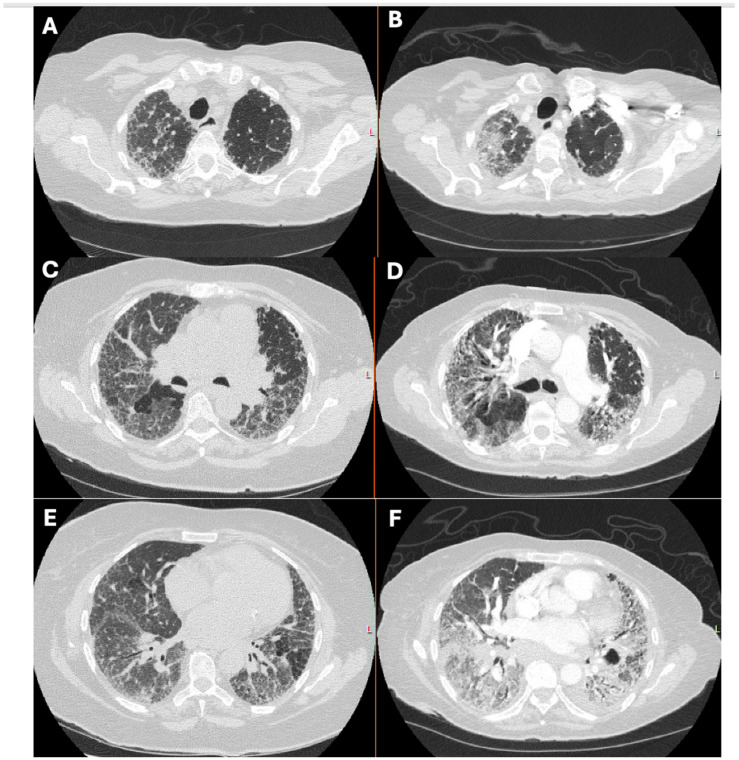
Chest CT images of a patient with ILD at baseline and with an acute exacerbation (a few months after). The images (A,C,E) show the baseline disease involving interstitial thickening, reticulations, and mosaicism, while the images (B,D,F) show pronounced ground-glass opacities and increased interstitial infiltrates, indicative of an exacerbation when the patient was in the ICU.
